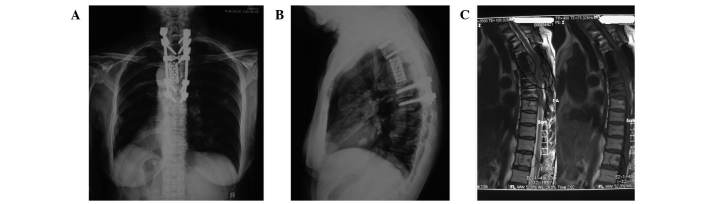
Image examination results of a patient two years after posterior vertebral column resection. (A) Anterior-posterior and (B) lateral X-rays of the thoracic spine two years after surgery; (C) magnetic resonance imaging of the thoracic spine two years after surgery showed local recurrence and a new lesion at T12 (original lesion located at T4 and T5).
